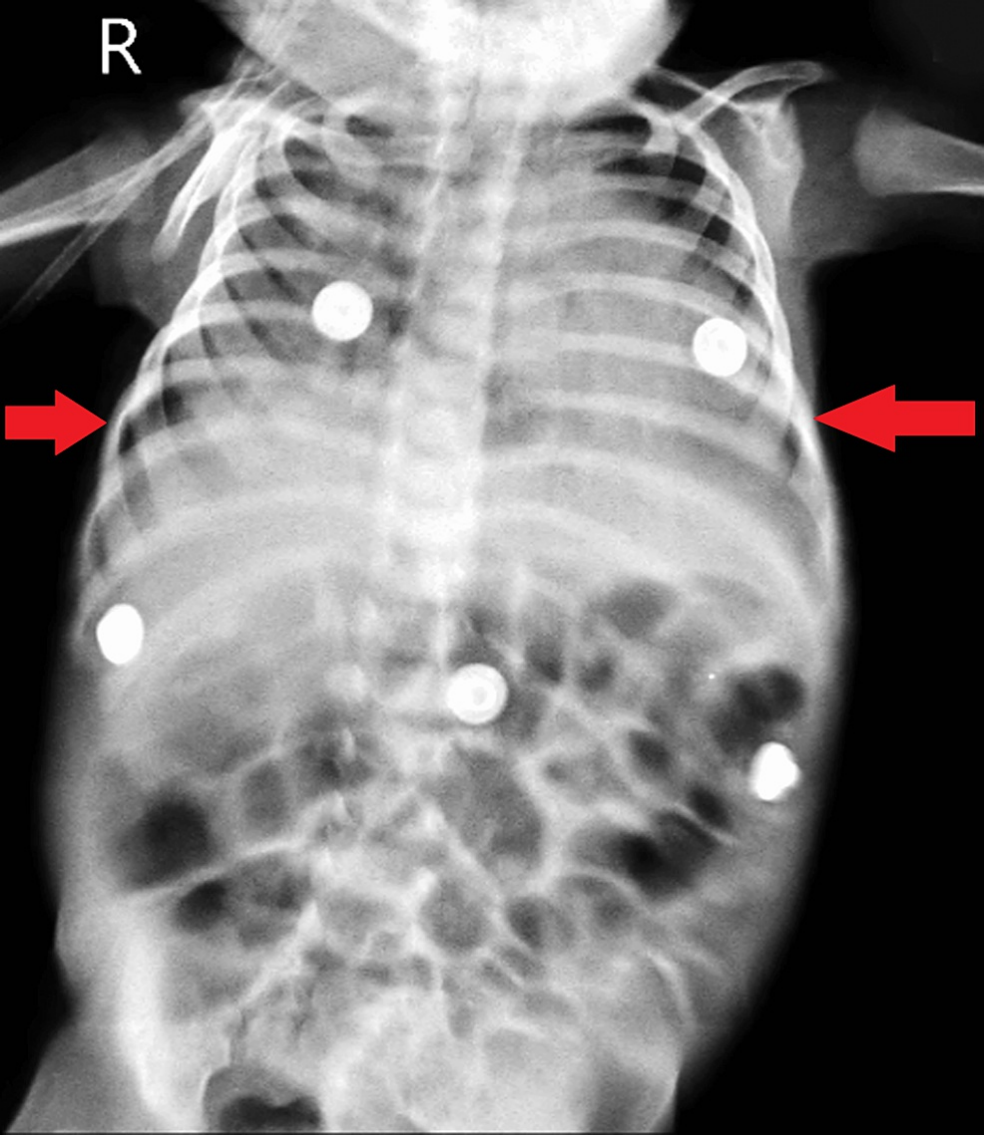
External chest X-ray showing bilateral patches and diffuse haziness on the right side.
